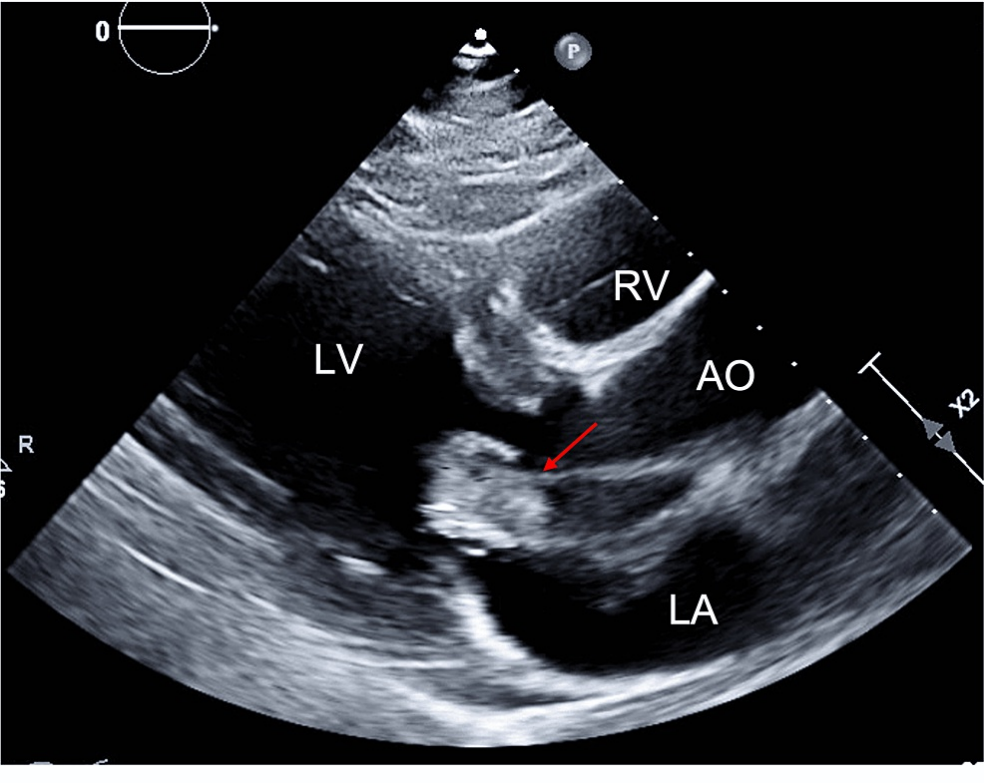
TTE with parasternal long-axis view demonstrating a 4.65 × 2 cm mass (red arrow) in the left atrium attached to the atrial septum. TTE: transthoracic echocardiogram
